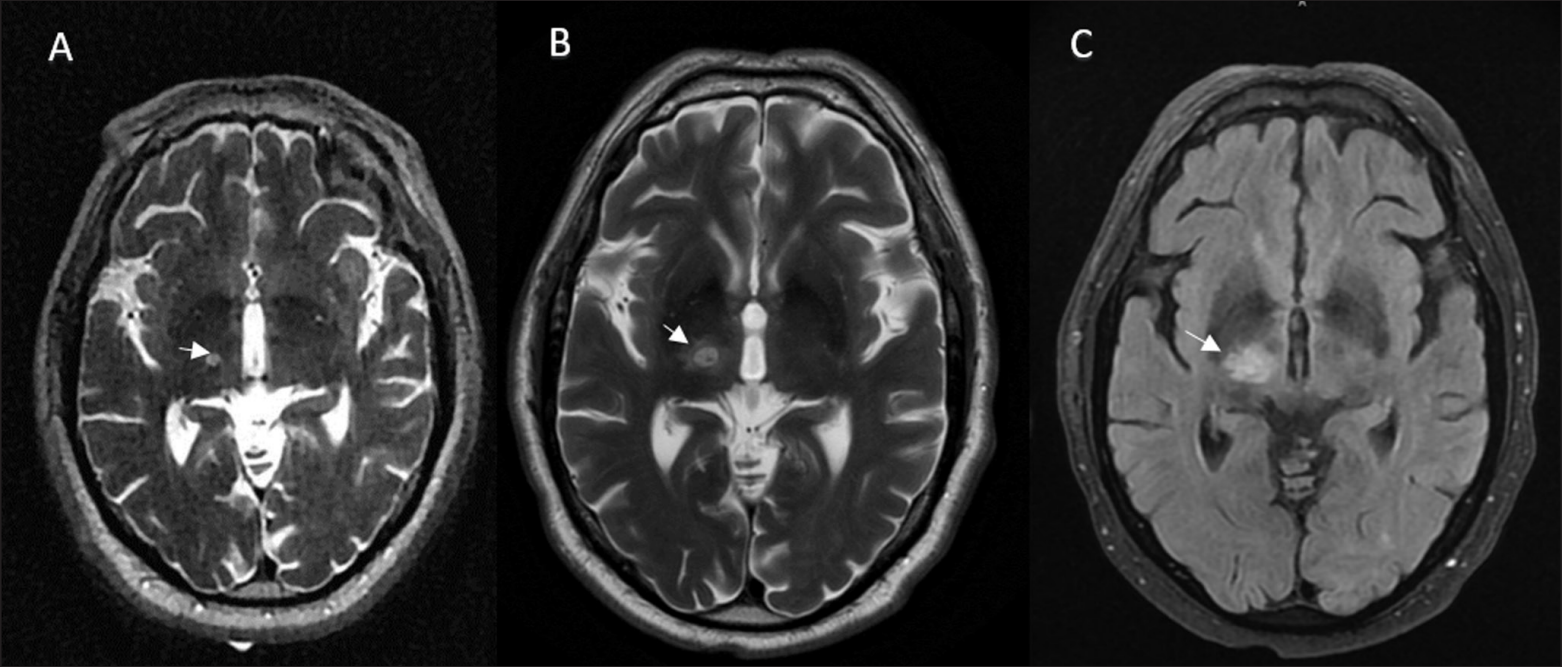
Left to right A. Postoperative day-0 (POD -0) – transverse T2 image with lesion in the right thalamus. B. POD-1 expansion of lesion seen on T2 sequence. C. POD-1, Fluid-attenuated inversion recovery (FLAIR) sequence demonstrating edema encroaching posterior limb of internal capsule.
